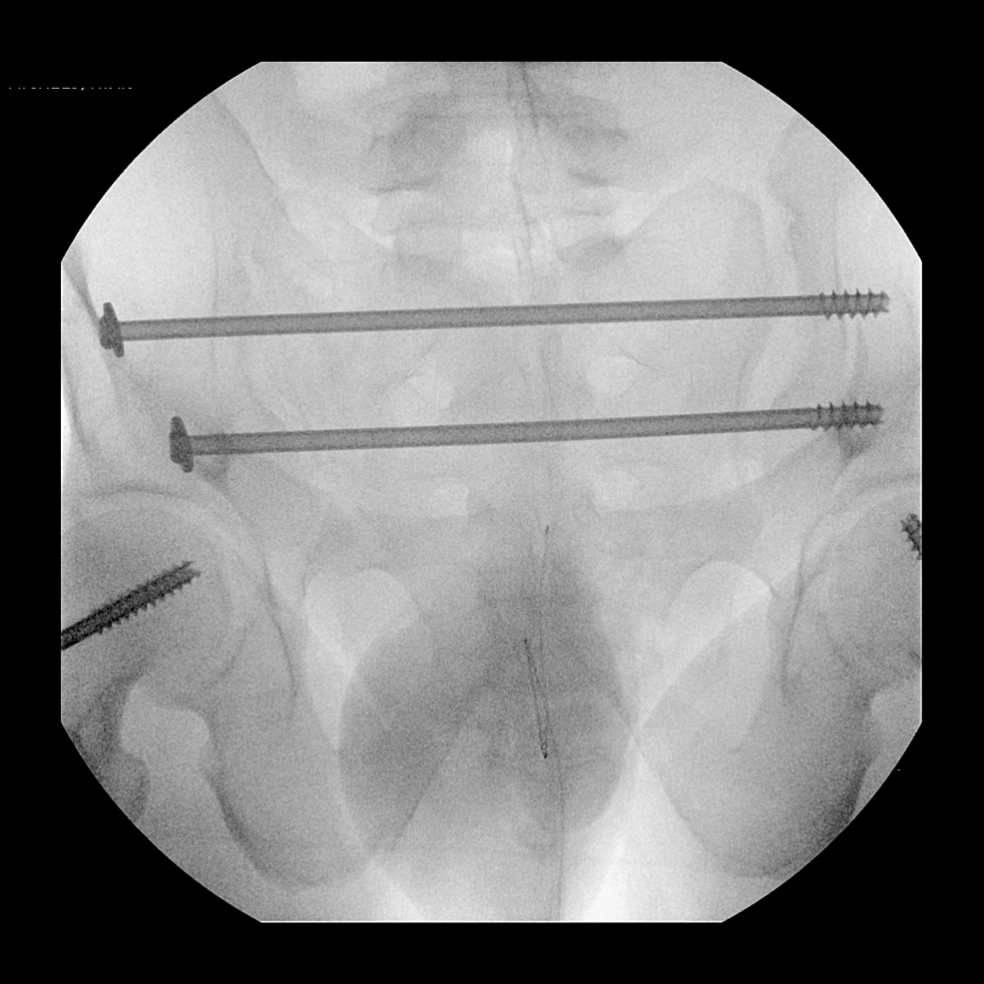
CT scan of the pelvis shows an ORIF of the fracture through the sacroiliac joint with postoperative anatomic alignment. ORIF: open reduction internal fixation
